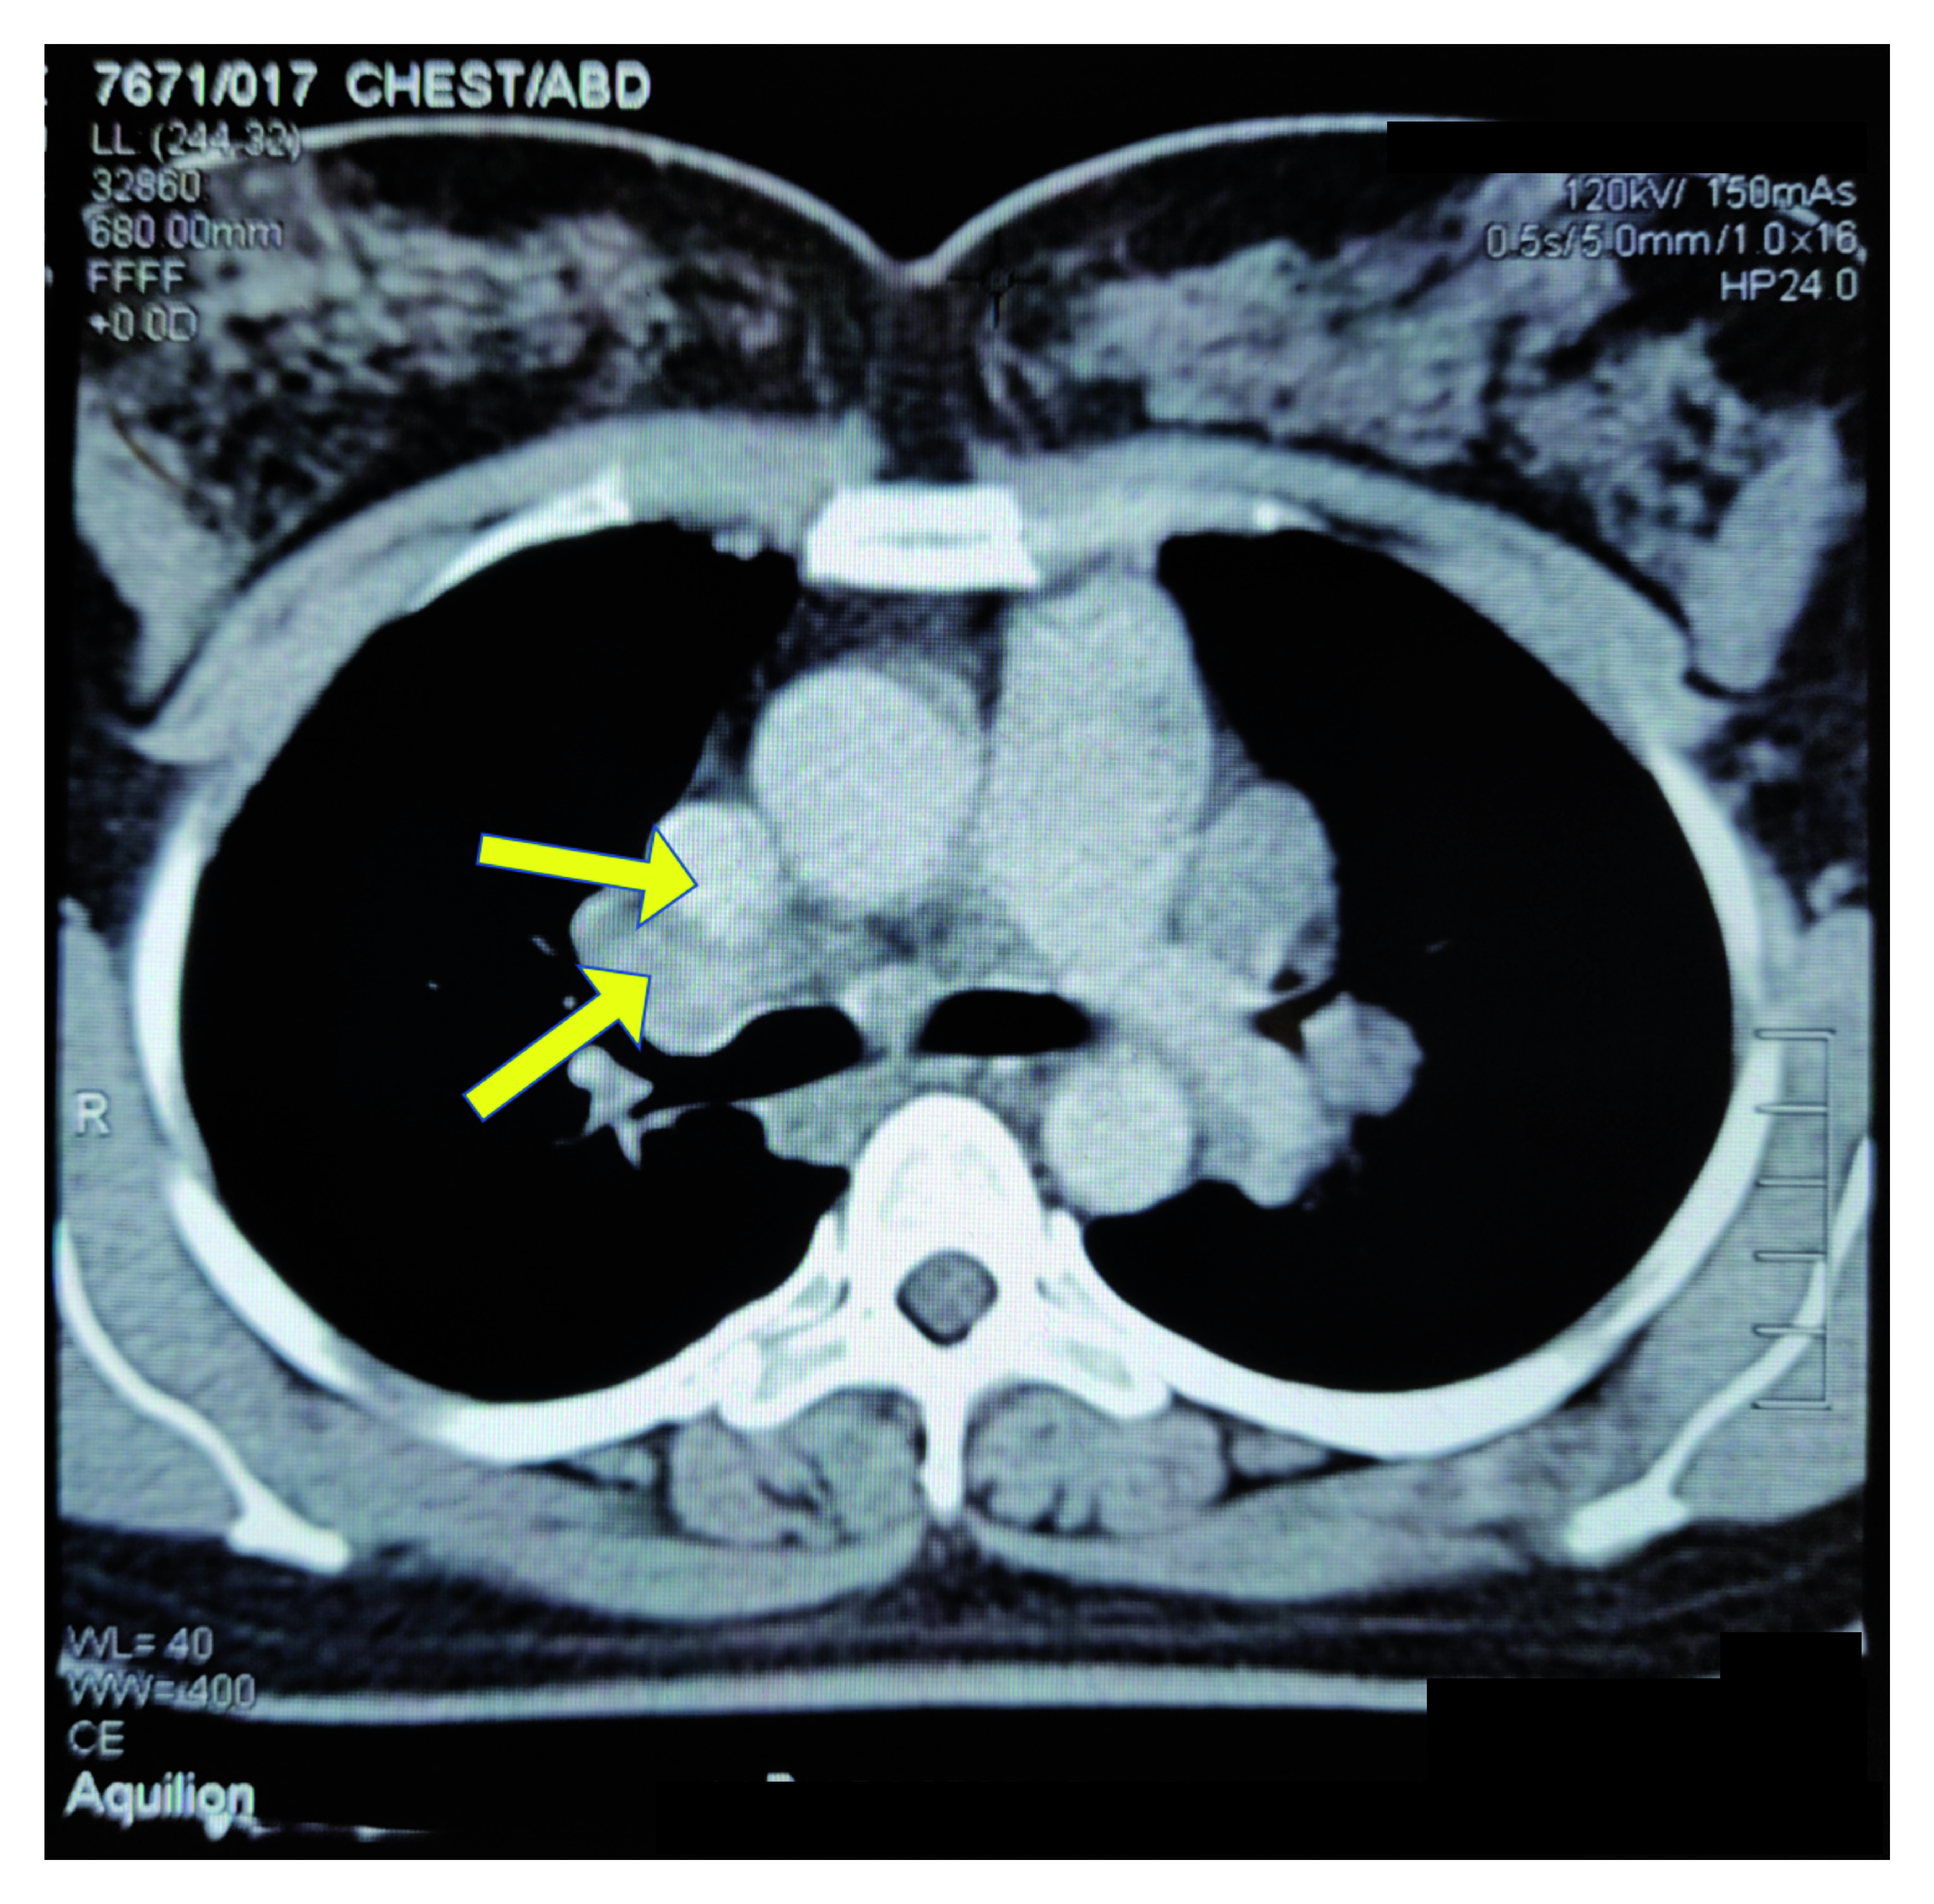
Preoperative chest computed tomography scan. Small (2.5 cm × 1.6 cm) soft tissue density mass with speculated margins in the upper quadrant of the left breast with possible focal infiltration of the underlying chest wall muscle is observed (shown by yellow arrows).
